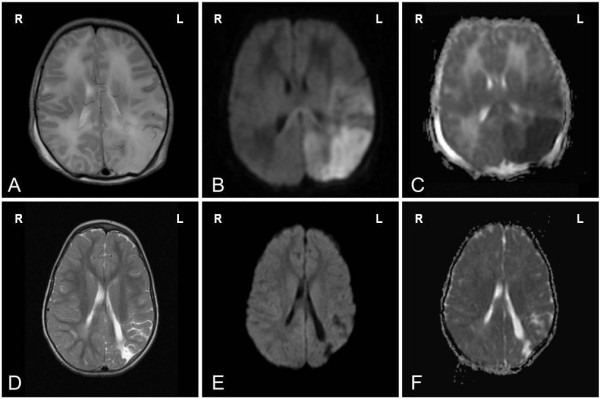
MRI of ischemic lesions. Abnormal signal changes in multiple brain areas due to ischemic lesions in the distribution of the middle and posterior cerebral artery on the left side. A-C: MRI on the 4^th^ postnatal day. A: High-signal intensity in T2w imaging corresponding to B: high-signal intensity in diffusion weighted imaging (DWI) (b1000) and C: low-signal intensity in apparent diffusion coefficient maps (ADC-MAP). D-F: MRI at 32 months. D: T2w imaging E: DWI.(b1000) and F: ADC-MAP showing now a scar formation on the left occipital lobe.
